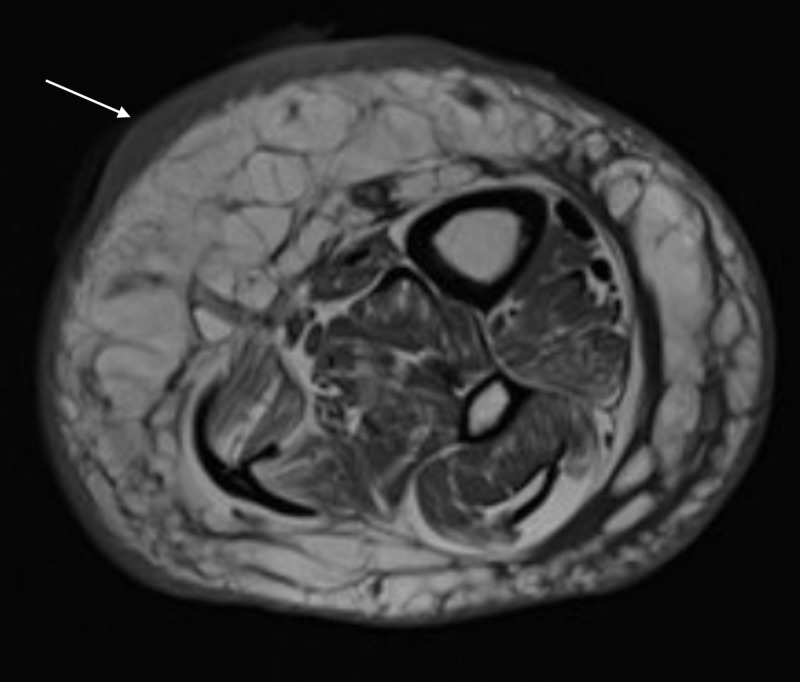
MRI showing wound (white arrow) medially in the distal calf with adjacent skin thickening along with circumferential subcutaneous edema MRI: magnetic resonance imaging
